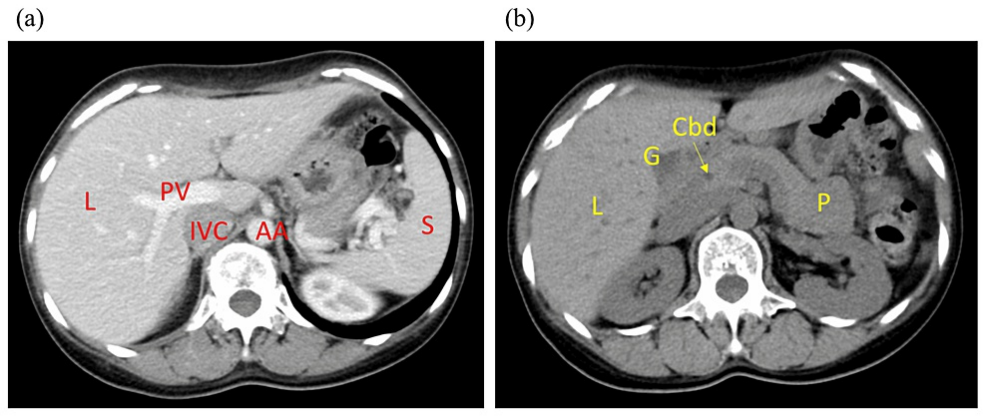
Abdominal computed tomography images (a) Contrast-enhanced image; (b) non-contrast image. Both images show no significant abnormal findings in the liver and biliary system, including the gallbladder and intra- and extrahepatic bile ducts. AA: abdominal aorta; Cbd: common bile duct; G: gallbladder; IVC: inferior vena cava; P: pancreas; PV: portal vein; S: spleen; L: liver
